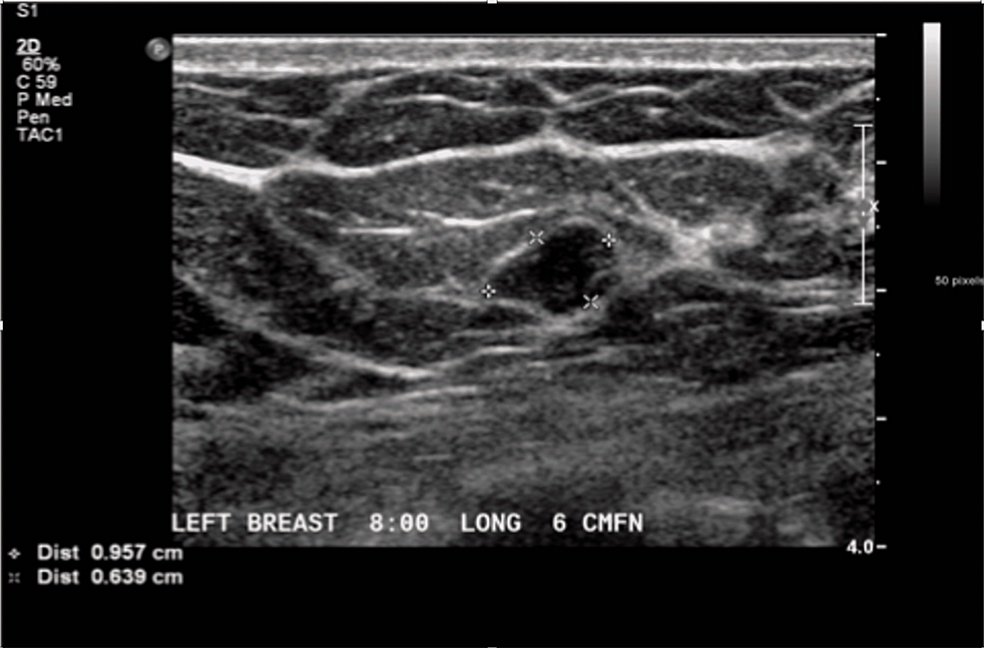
Breast ultrasound demonstrates a well-defined ovoid hypoechoic 1 cm nodule.
